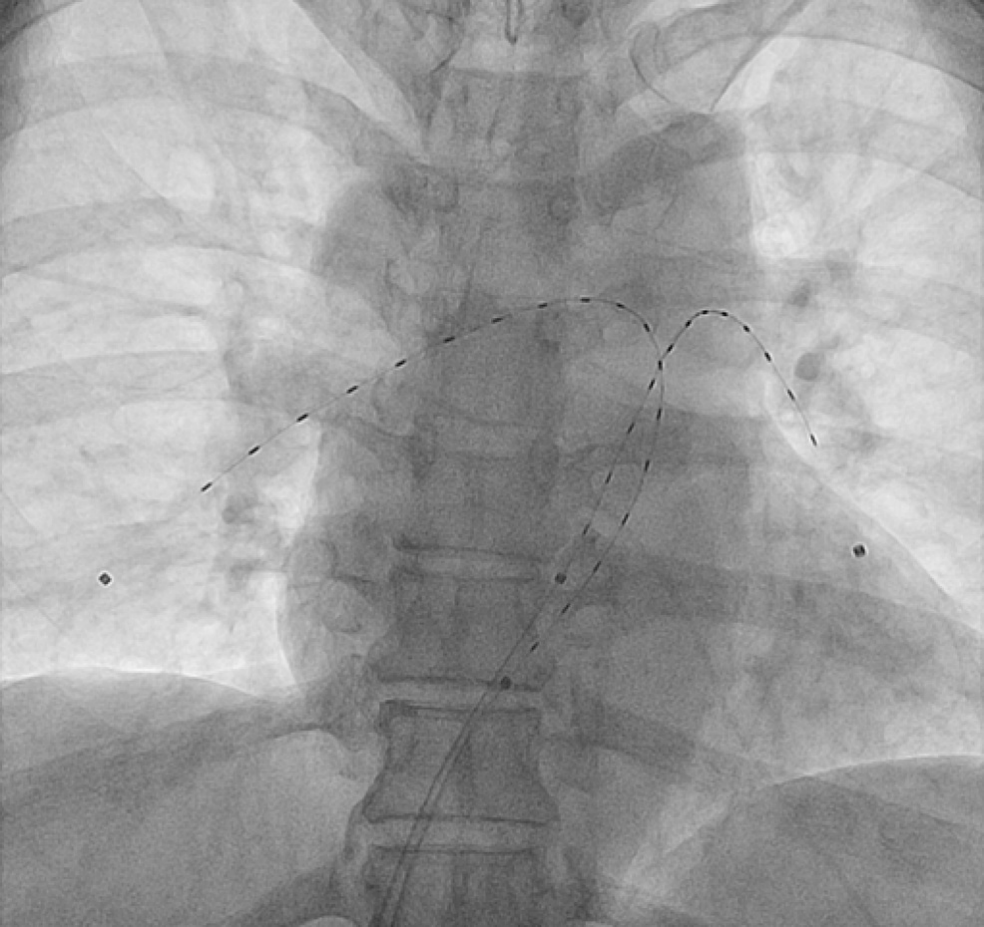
EKOS ultrasound image of the patient EKOS: EkoSonic™ Endovascular System
